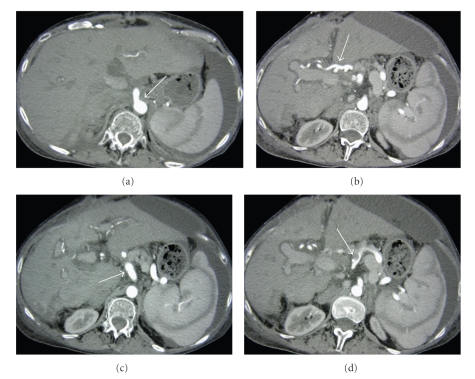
(a) Axial source image from CT angiogram (CTA) demonstrates donor hepatic artery anastomosed to the proximal aorta (arrow). Note normal arterial perfusional striations in the spleen. (b) Axial CTA source image shows the donor hepatic artery coursing to the hepatic hilum (arrow). (c) Axial CTA source image shows thin, transversely oriented intimal flap from an arterial dissection in the recipient celiac trunk (arrow). (d) Axial CTA source image depicts the initmal flap of the celiac trunk dissection extending into the splenic artery. False lumen thrombus in the partially occluded splenic artery is apparent (arrow).
